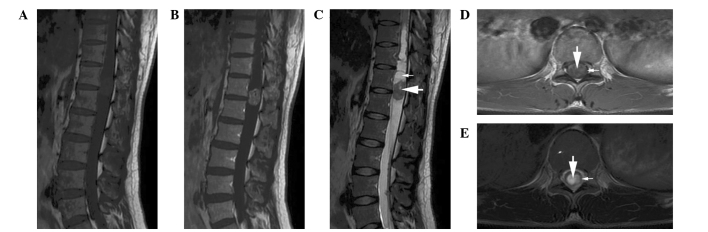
Pre-operative magnetic resonance imaging. (A) Sagittal T1-weighted image revealing an intramedullary cystic-solid lesion in the conus medullaris. (B) Solid lesion demonstrating inhomogeneous enhancement following gadolinium injection. (C) T2-weighted image revealing the solid mass (large arrow) accompanied by a cranial cystic lesion (small arrow). (D) Coronary contrast-enhanced T1-weighted image showing the solid mass (large arrow) located exclusively within the conus medullaris (small arrow). (E) Coronary T2-weighted image indicating the additional cranial cystic lesion (large arrow) present in the spinal cord (small arrow).
